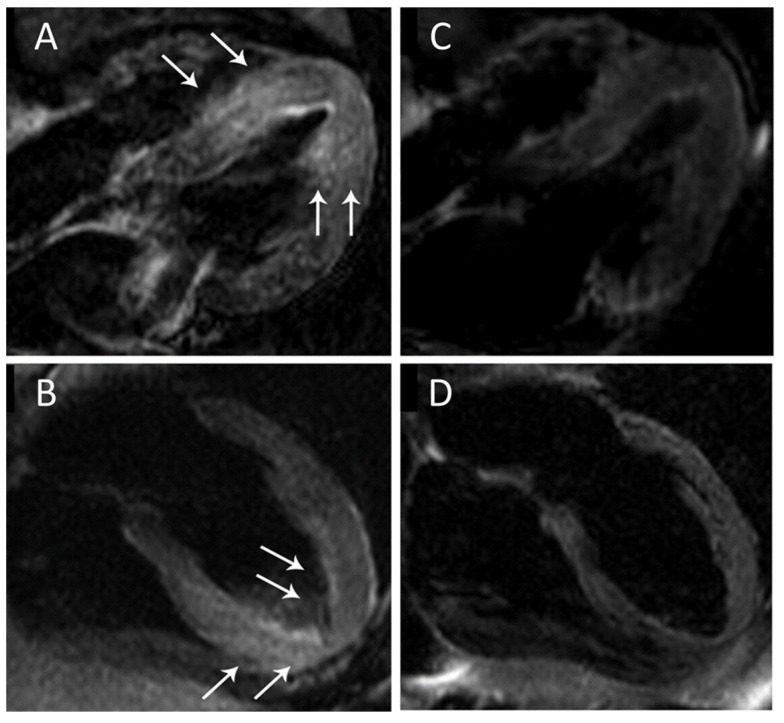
T2-weighted sequences showing myocardial edema during acute phase (left) and follow-up (right). Four-chamber view showing edema in the left ventricular mid and apical inferoseptal and anterolateral segments (arrows in (A,B)). T2-weighted sequences on follow-up scan showing disappearance of edema (C,D). Adapted with kind permission from Migliore et al. [42].
